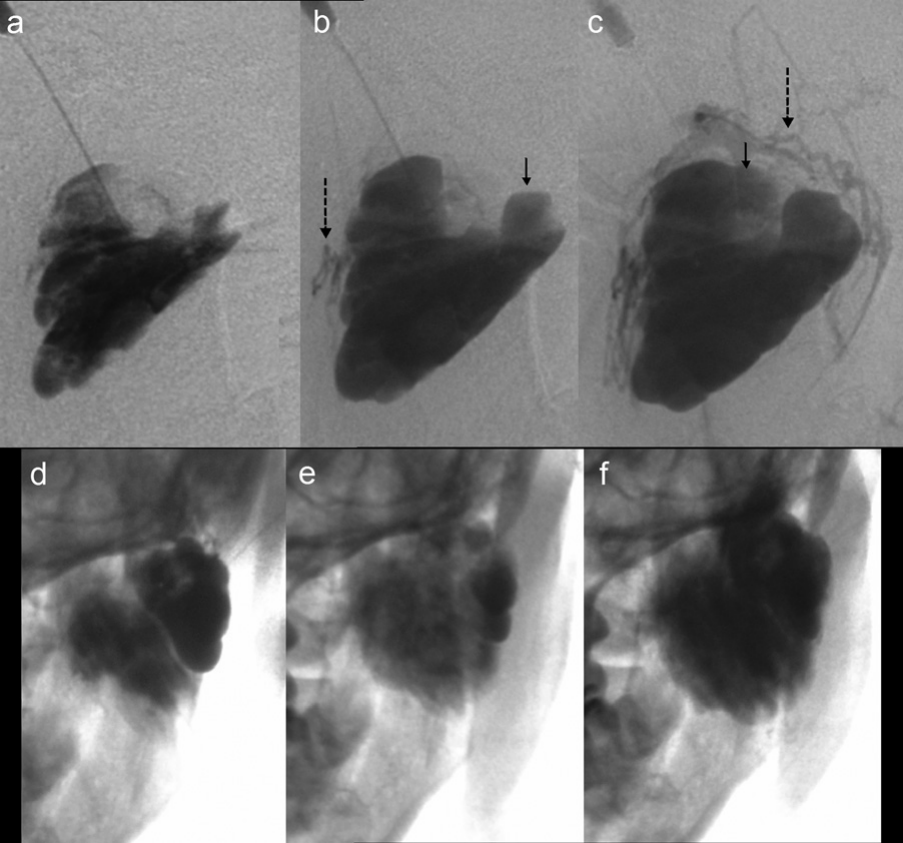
Intramuscular and extramuscular cisterns. Cisternography in a young woman who began experiencing pain in the upper extremity at the end of the first trimester. The ‘bunch of grapes’ pattern is seen around the clavicle (a), and an elongated cystic pattern is visible in and along the fascicles in the pectoralis major muscle (PM) (b). Appearance of the drainage vessel was faster at the PM aspect (not shown: continuous injection was terminated because of pain). MRI images (c–e) show continuity of the cisterns, with subclavicular cisterns showing continuity with those on the trapezius muscle and the PM. Symbols: white arrow, cisterns in the PM; dotted white arrow, cisterns in the trapezius muscle
